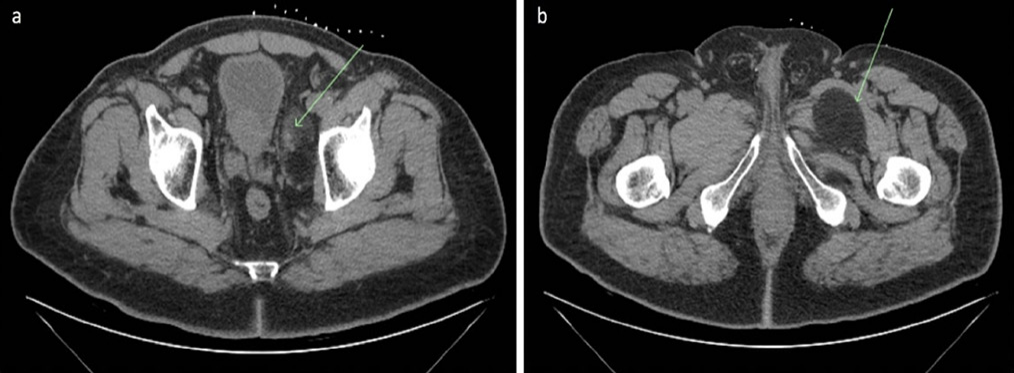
(a – b) Axial CT images of the biopsy tract targeted at the area of enhancement within the upper soft tissue nodule and the septations within the lower border of the lesion.
